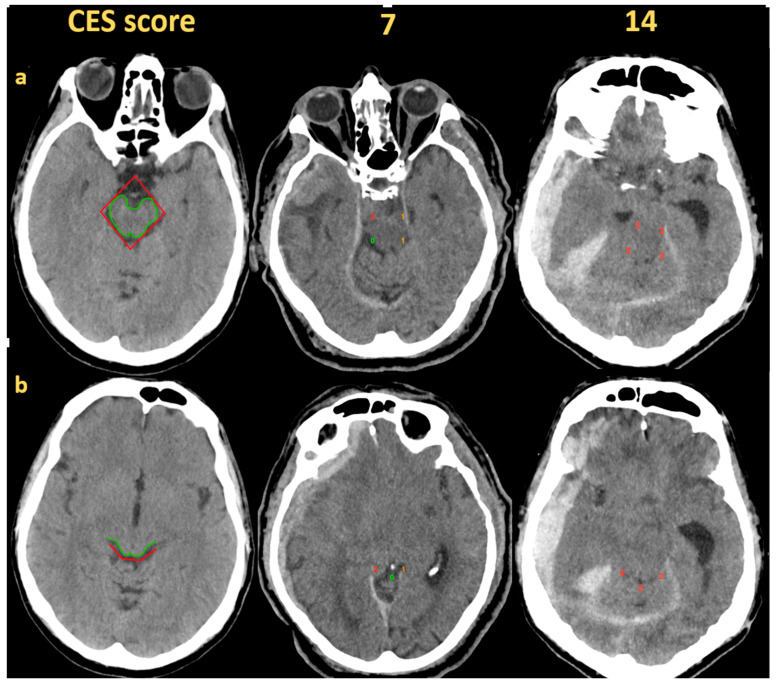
Cisternal effacement score evaluation is based on CT axial cuts at the level of the perimesencephalic (upper row, a) and quadrigeminal cisterns (lower row, b). The areas marked with the red color represent cisternal components used to calculate the cisternal effacement score. Two cases with CES of 7 and 14 are presented as an example.
